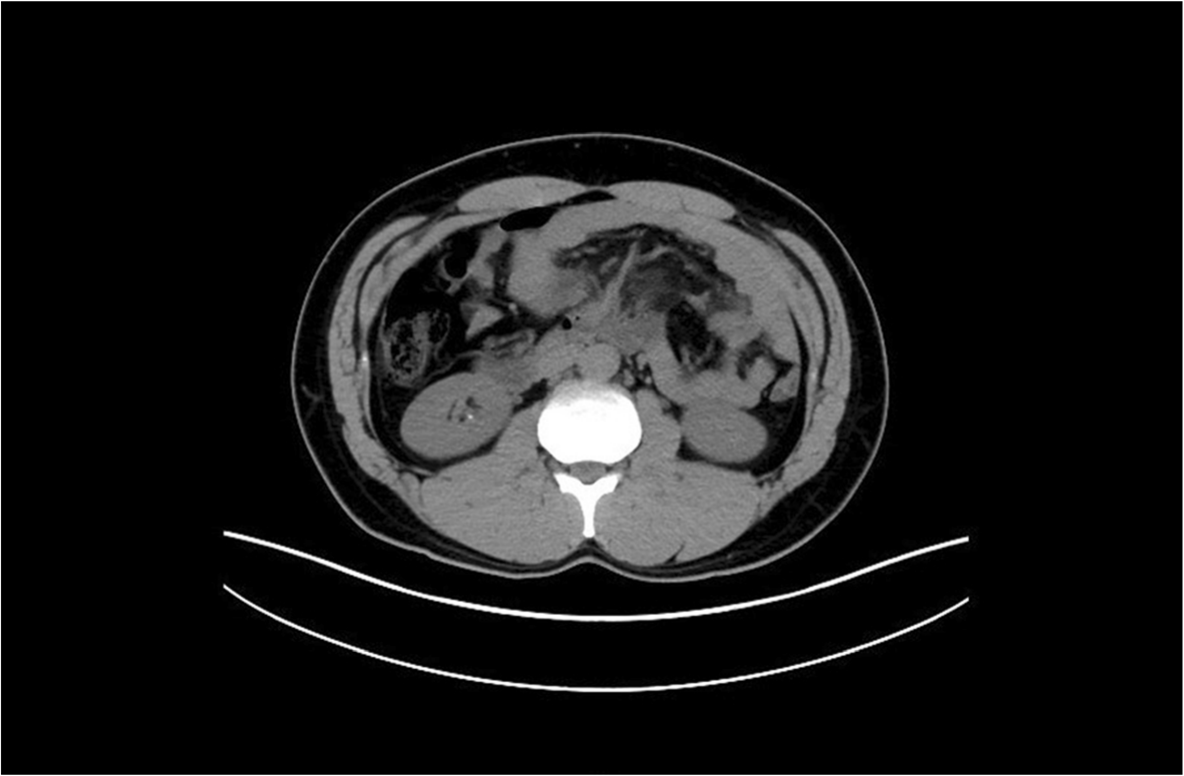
Whole abdominal CT of patients. CT image showed small stones in his right kidney
